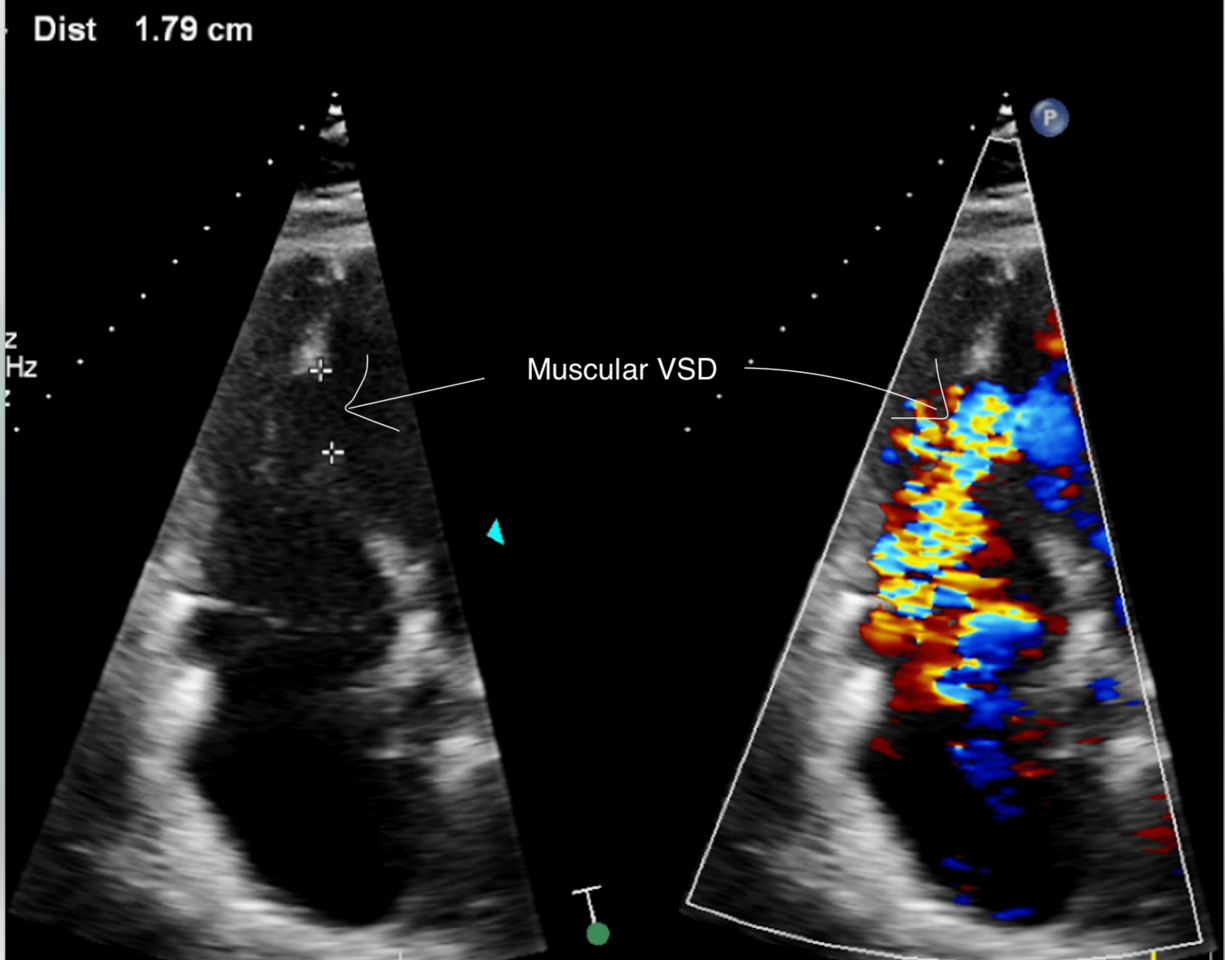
TTE without and with color flow showing muscular VSD measuring 1.79 cm TTE: transthoracic echocardiography, VSD: ventricular septal defect
